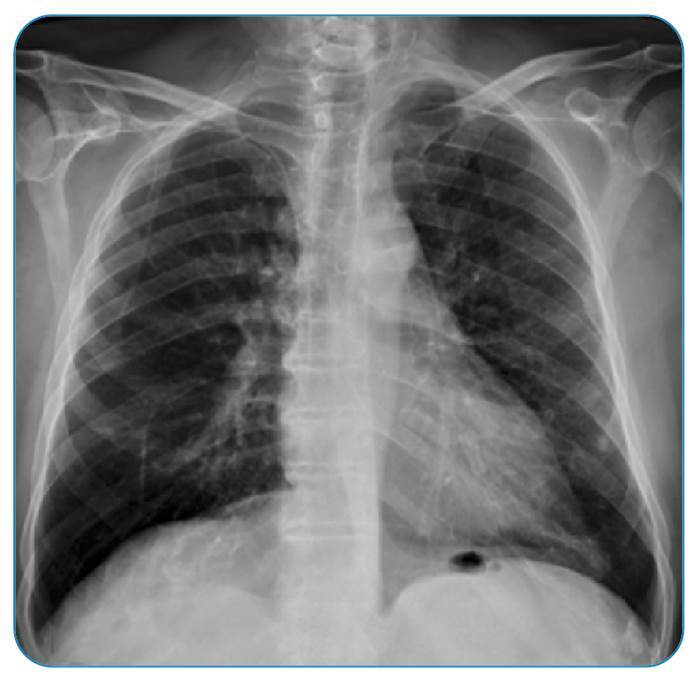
Chest radiograph after 10 days of trimethoprim-sulfamethoxazole treatment.
